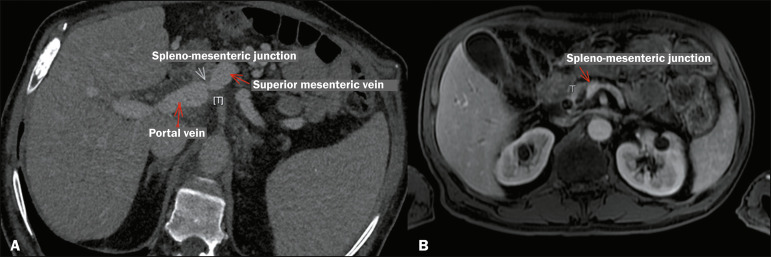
Axial contrast-enhanced CT scan of the abdomen (A), in the portal
